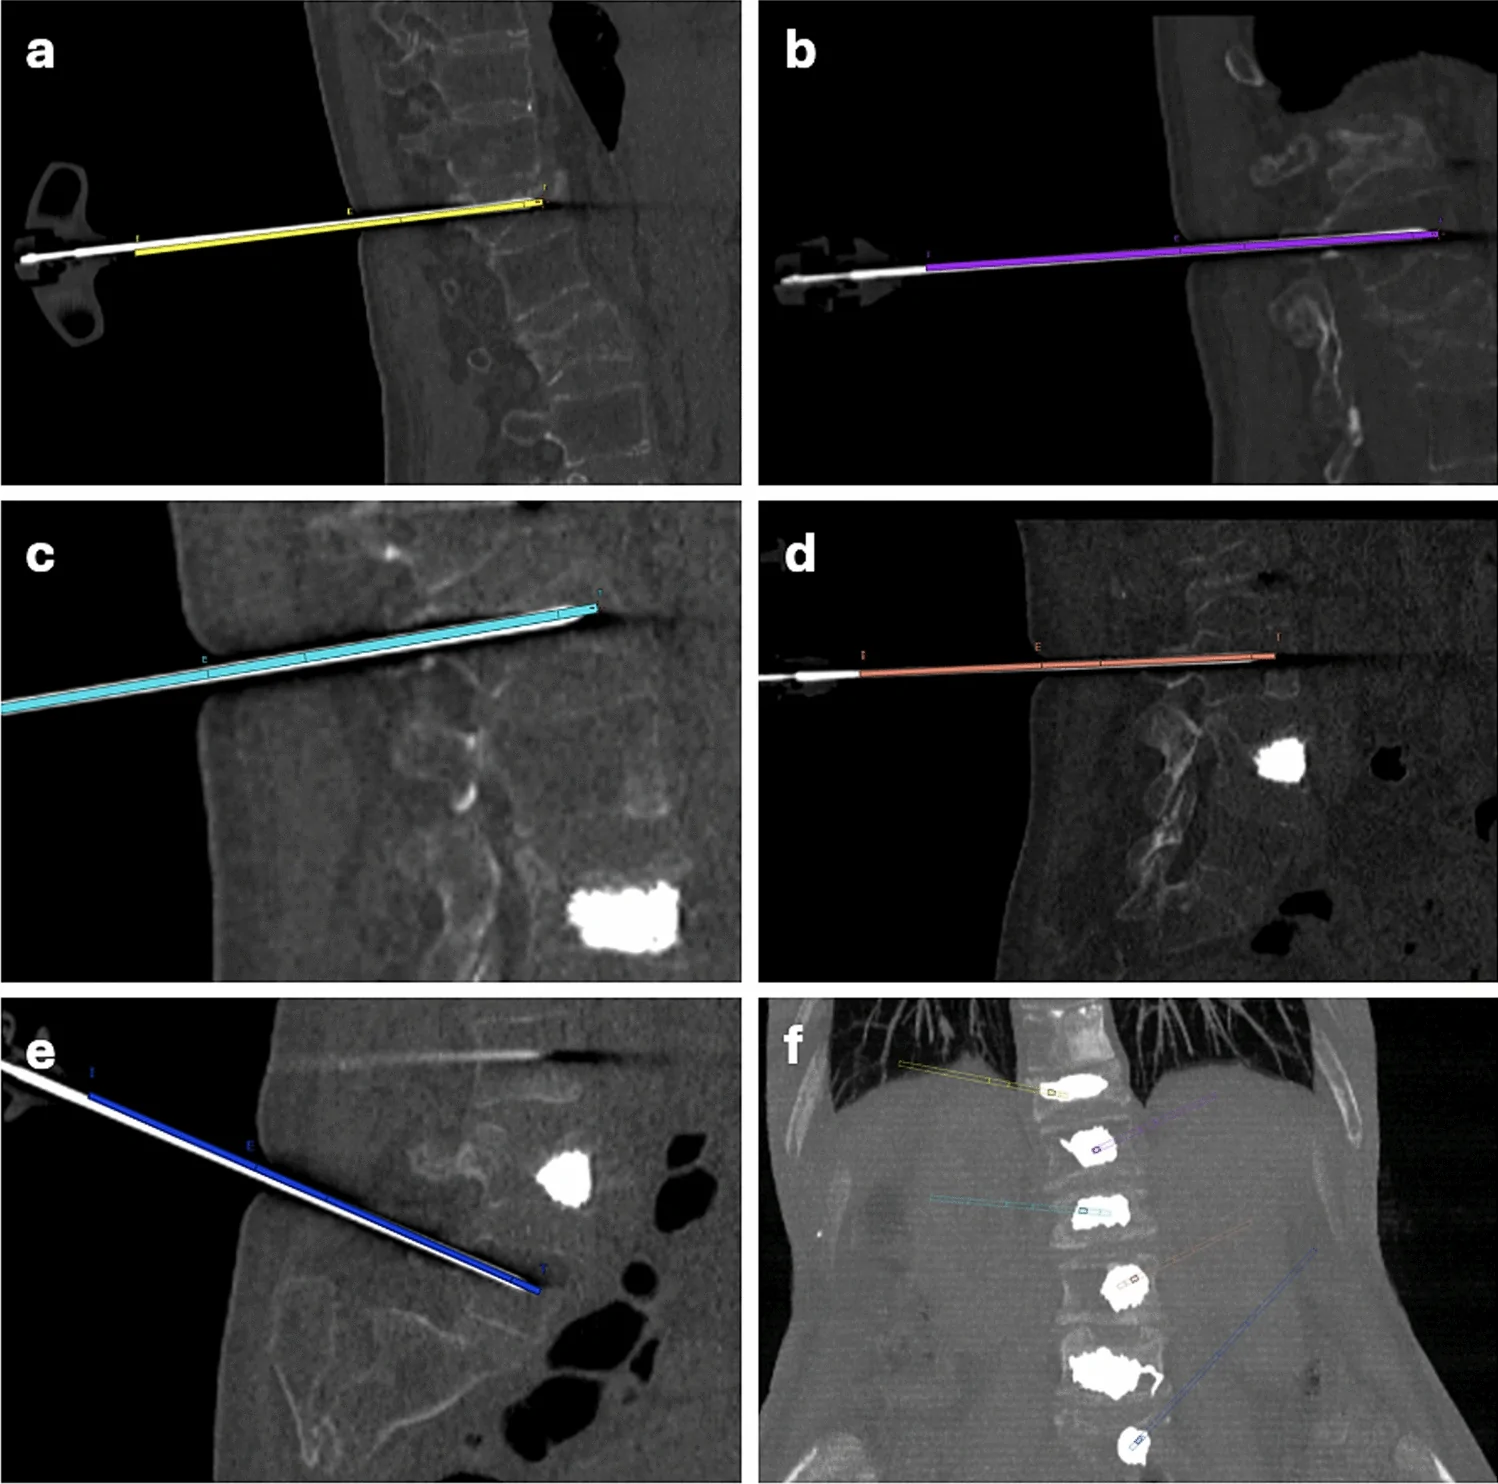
Representative case of multilevel vertebral consolidation. A 49-year-old woman with metastatic bone disease from a thymic neuroendocrine tumor presented with multiple thoracolumbar vertebral fractures involving T12 to L5, associated with mixed metastatic and osteoporotic involvement and severe disabling pain. The patient had previously undergone vertebroplasty at L4 and presented with new compression fractures at T12-L3 and L5. Multilevel vertebroplasty was performed for analgesic and consolidation purposes. (a-e) Intraoperative oblique sagittal CT images obtained in the plane of the pedicular trajectories at T12-L3 and L5, with superimposed planned robotic trajectories (colored lines). (f) Coronal maximum intensity projection reconstruction from the post-procedural CT showing satisfactory cement distribution across the treated vertebral bodies
